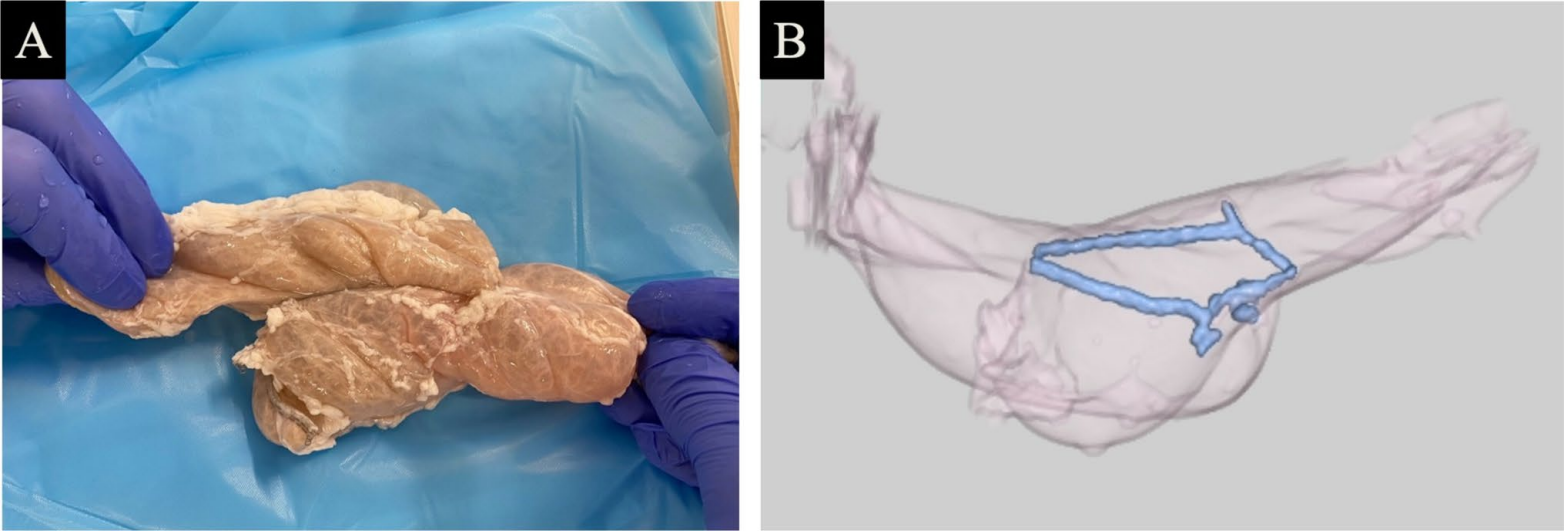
A The completed overlap anastomosis is filled with 20 ml of saline solution mounted on a fixed table and imaged using maximum intensity projection-computed tomography (MIP-CT). B The anastomotic area was measured by plotting and tracing a temporary line between the cut edges of the staple line in 1-mm increments with MIP-CT
